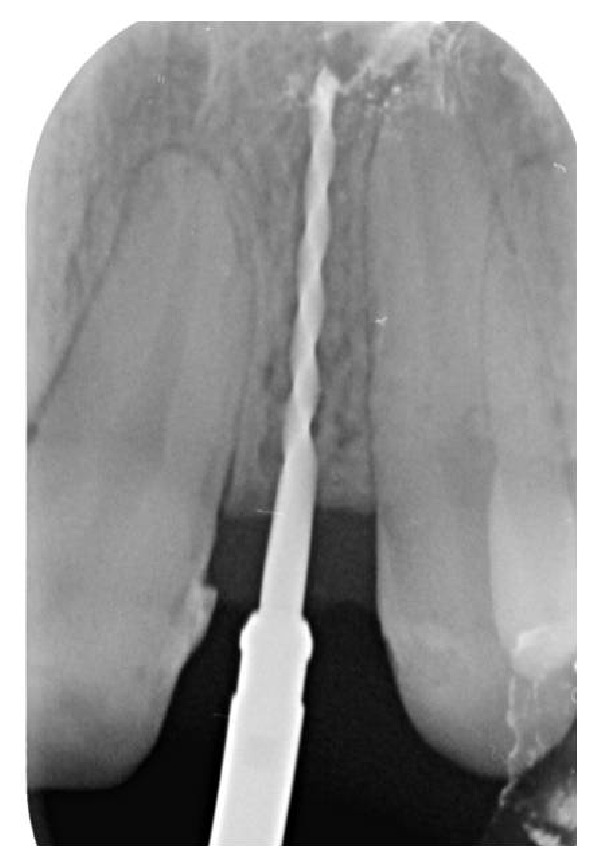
Radiographic examination of the correct inclination in site to the right.
